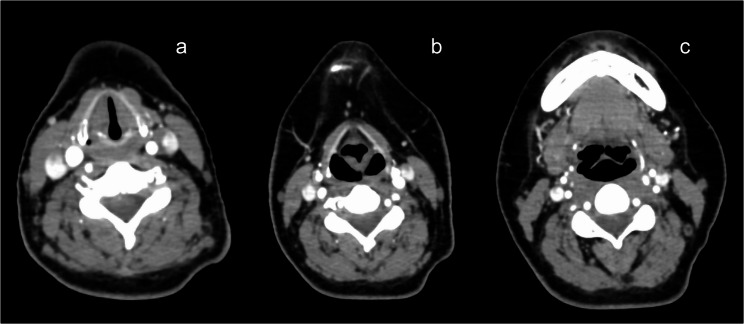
Preoperative computed tomography images of the neck (a glottic level, b arytenoid level; c epiglottic level): no apparent anatomical abnormalities of the larynx can be found
